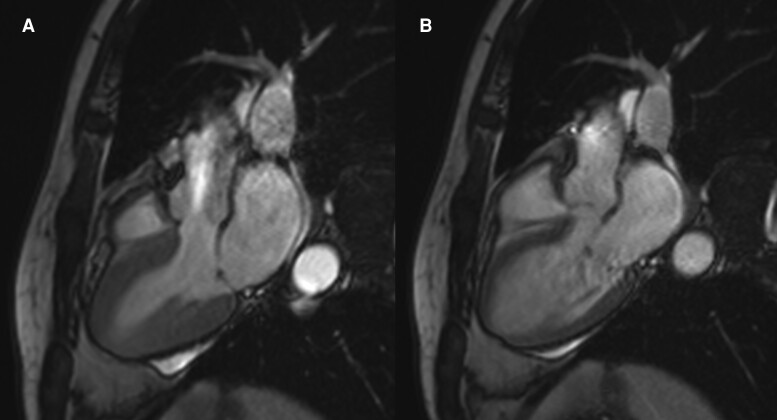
Cardiac MRI at three-month follow-up. A short-axis view is shown in end-systole (*A*) and end-diastole (*B*), revealing the absence of morphological abnormalities and apical dyskinesia.
